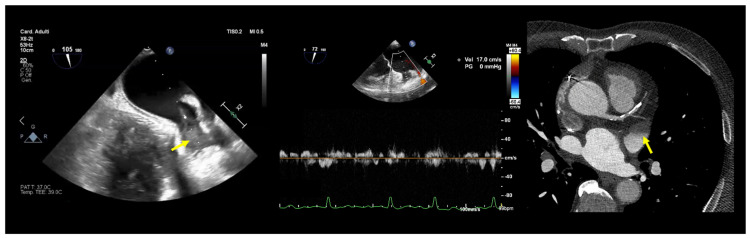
TEE in a patient with transthyretin cardiac amyloidosis: presence of left atrial appendage thrombus (arrow) and severe reduction in left atrial appendage pulsed-wave Doppler emptying velocity. Cardiac CT of the same patient, performed during follow-up, con-firming the presence of a stratified left atrial appendage thrombus (arrow).
